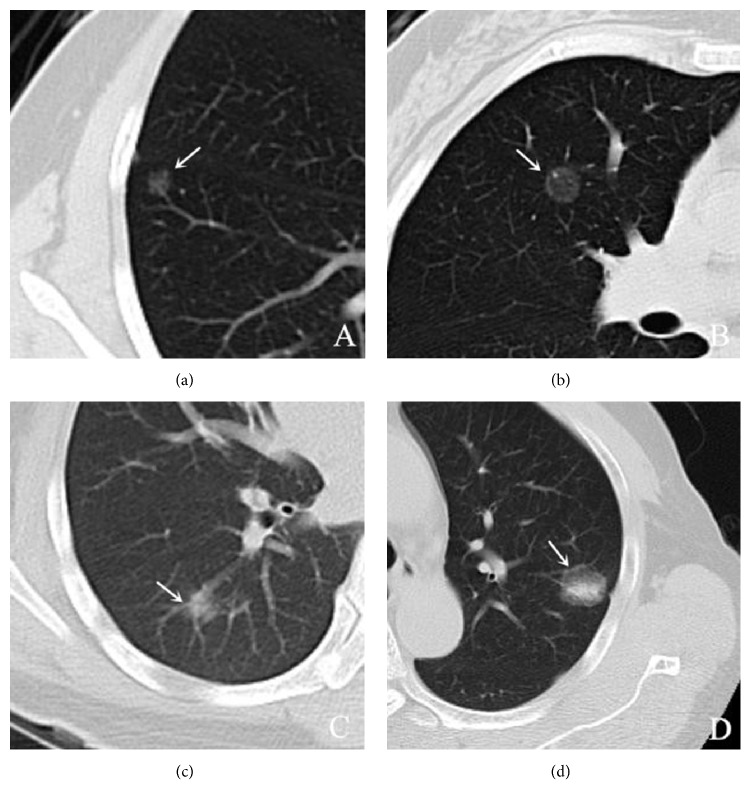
CT images show preinvasive lesions and invasive adenocarcinomas. (a) A 7 mm mGGN (arrow) is shown in the anterior segment of the right upper lobe in a 53-year-old woman. This nodule was confirmed pathologically as a preinvasive lesion (AAH). (b) A 13 mm mGGN (arrow) is shown in the anterior segment of the right upper lobe in a 53-year-old woman. This nodule was confirmed pathologically as a preinvasive lesion (AIS). (c) An 11 mm mGGN (arrow) is shown in the posterior segment of the right upper lobe in a 35-year-old woman. This nodule was confirmed pathologically as an invasive pulmonary adenocarcinoma (MIA). (d) A 21 mm mGGN (arrow) is shown in the posterior segment of the right upper lobe in a 35-year-old woman. This nodule was confirmed pathologically as an invasive pulmonary adenocarcinoma (IAC). Note: AAH=atypical adenomatous hyperplasia; AIS=adenocarcinoma in situ; MIA=minimally invasive adenocarcinoma; IAC=invasive adenocarcinoma.
